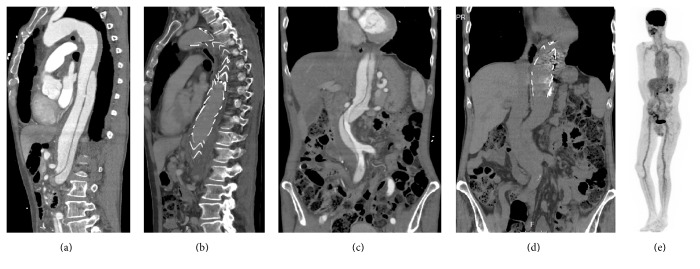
Radiological investigations. Sagittal ((a) and (b)) and coronal ((c) and (d)) reconstructions of CT scans, demonstrating type B aortic dissection and elongated aorta before and after TEVAR. The entry tear is demonstrated at the level of thoracic 10. 18-Fludeoxyglucose PET scan (e), demonstrating large vessel vasculitis.
